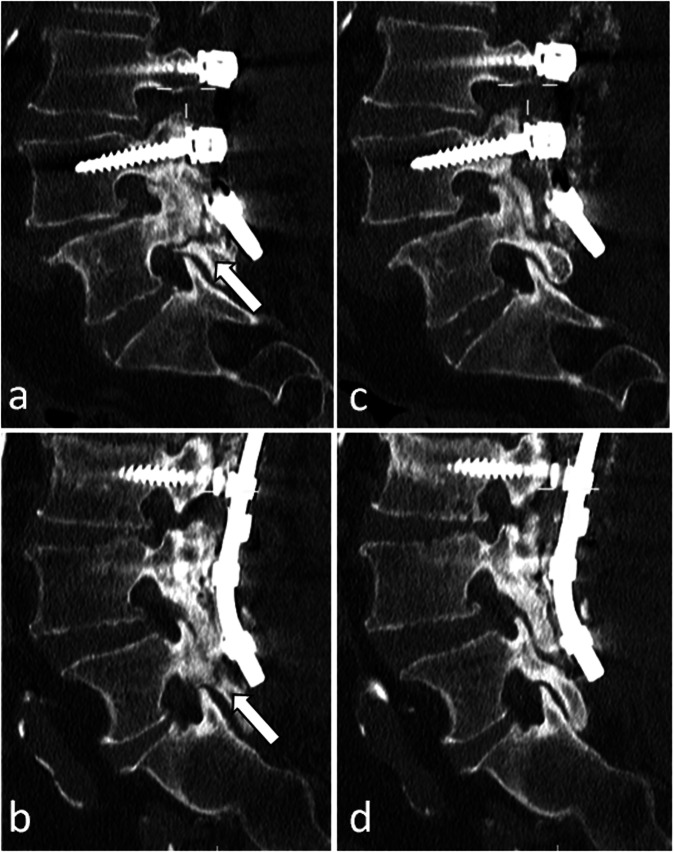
This patient complained of low back pain recurrence one year after a successful lumbar arthrodesis. On sagittal CT reconstructions (a, b) a bilateral pars articularis fracture is visible, which was unseen on a previous CT scan (c, d). This was attributed to an excessive stress on the posterior elements of the lumbosacral junction due to an incorrect restoration of the lordosis
